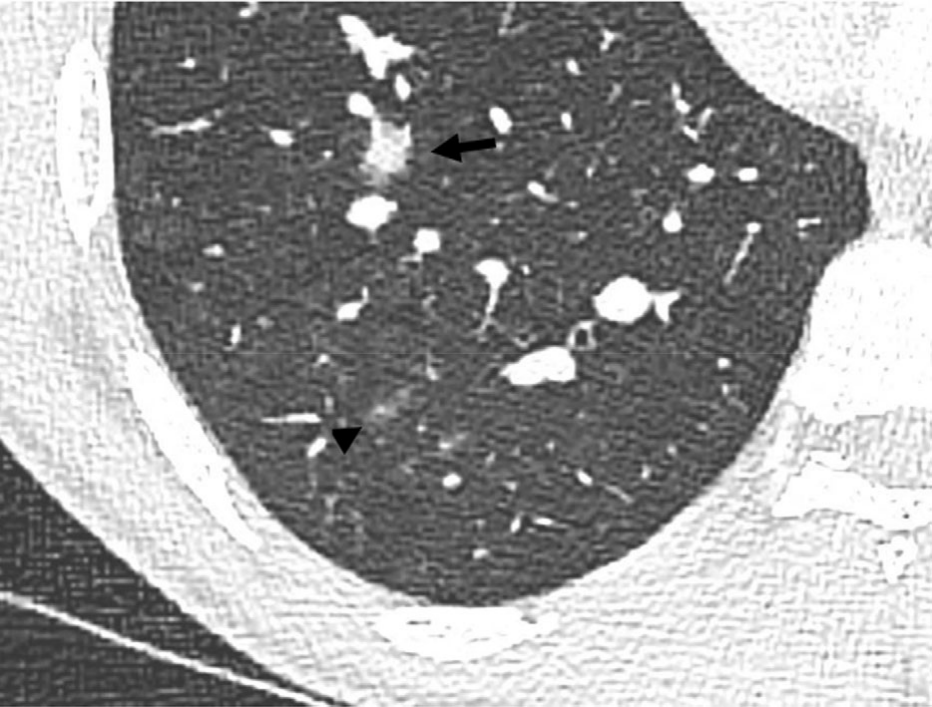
High resolution CT chest in a 24‐year‐old male patient diagnosed with GPA showing both irregular and ill‐defined centrilobular solid (arrow) and ground‐glass nodules (arrowhead), compatible with vasculitis.
